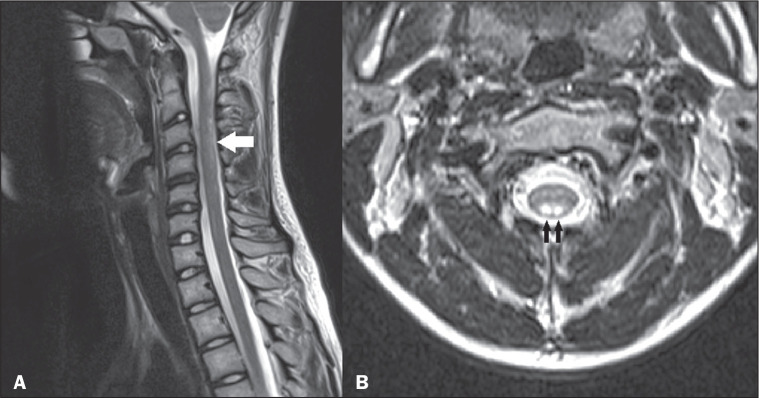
Vitamin B12 deficiency. Sagittal and axial T2-weighted MRI sequences (A
